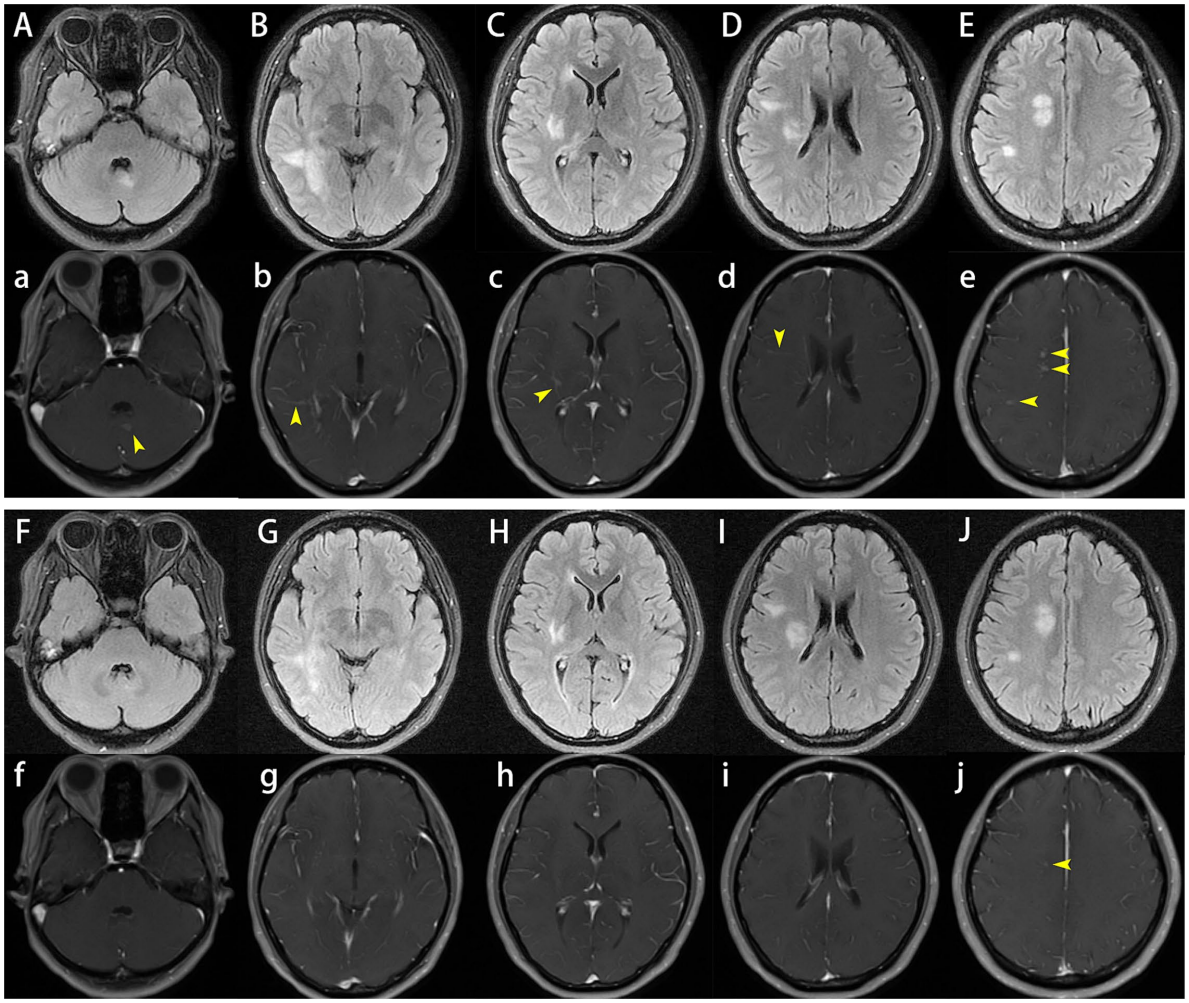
Cranial plain and enhanced magnetic resonance imaging (MRI). On admission, brain MRI demonstrated multiple abnormal signals in the intracranial region and cervical spinal cord, with nodular, patchy, or irregular enhancement (A–E,a–e), the central vein sign was observed on T1-weighted enhanced sequences (b–d). One month later, follow-up brain MRI showed that reduced lesion size and significant reduction or disappearance of enhancement (F–J,f–j).
